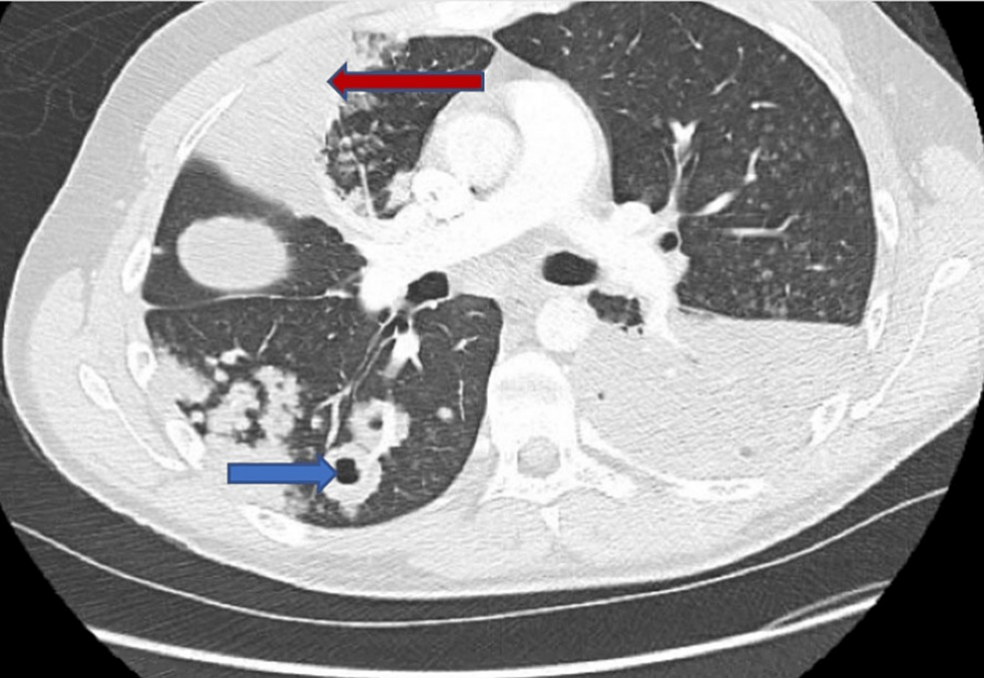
Computed tomography chest (axial images) The red arrow shows consolidation and the blue arrow shows evolving abscess.
